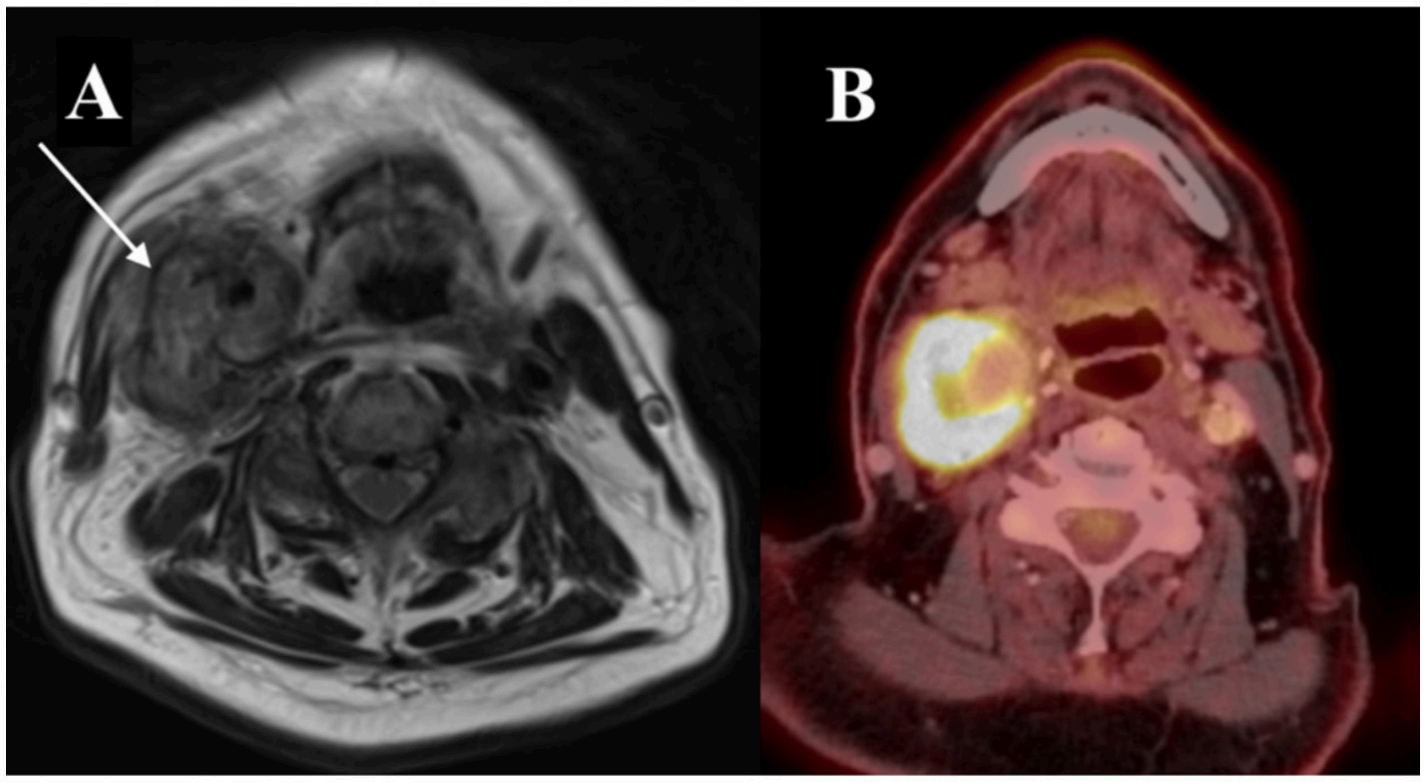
Initial diagnostic imaging. (A) MRI of the neck in the transverse plane. Tumor marked with an arrow. (B) PET/CT in the transverse plane showing uptake in relation to a slightly dilated right CCA.
